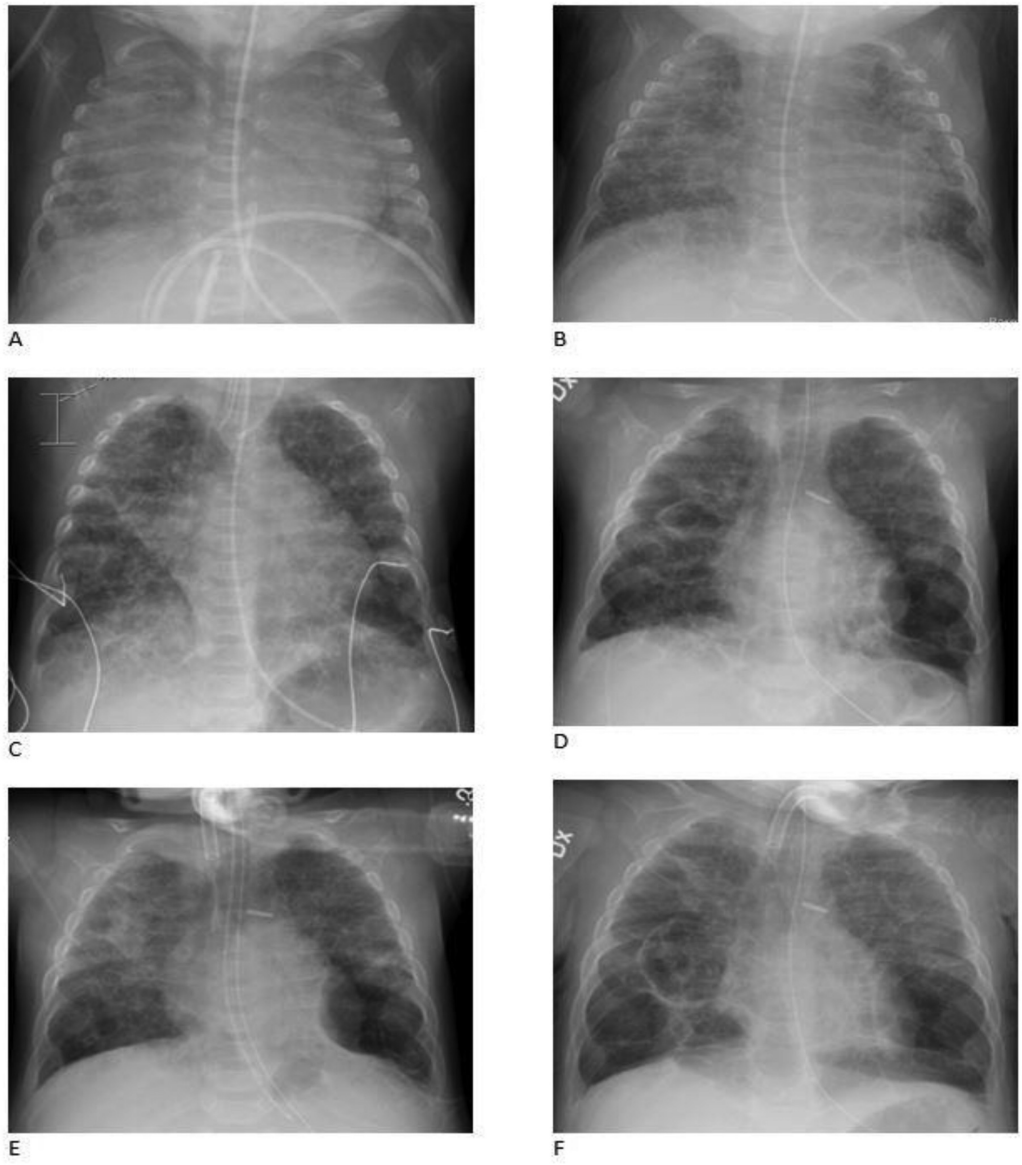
Representative chest radiographs. (A) PMA (post-menstrual age) 26w, (B) PMA 35w, (C) PMA 41w (before closure of the shunts), (D) PMA 50w (before tracheostomy), (E) PMA 55w (after tracheostomy) and (F) PMA 56w. The child developed early bilateral fine granular opacities in the pulmonary parenchyma and air bronchograms as seen in respiratory distress syndrome (RDS). Pulmonary edema could not be excluded. The granular opacities remained and became coarse. Hyperinflation and cystic changes developed and the cystic structure in left lower lobe was defined as pneumatocele. Transient apical atelectasis and non-specific parenchymal opacities occurred mainly on the right side.
